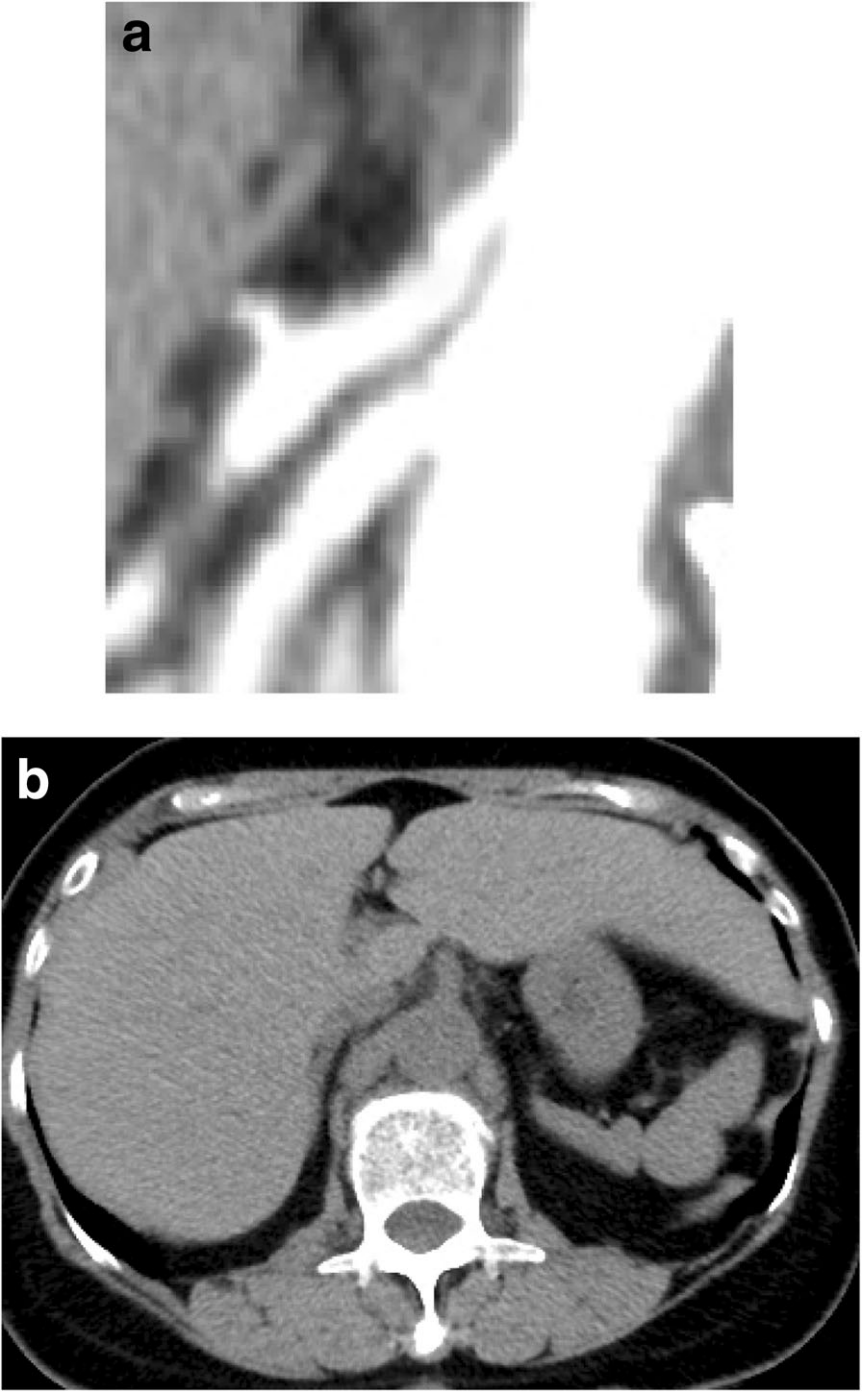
Preoperative multidetector CT showing neither median arcuate ligament syndrome nor arteriosclerosis around the celiac artery. a Contrast-enhanced CT. b Plain CT
